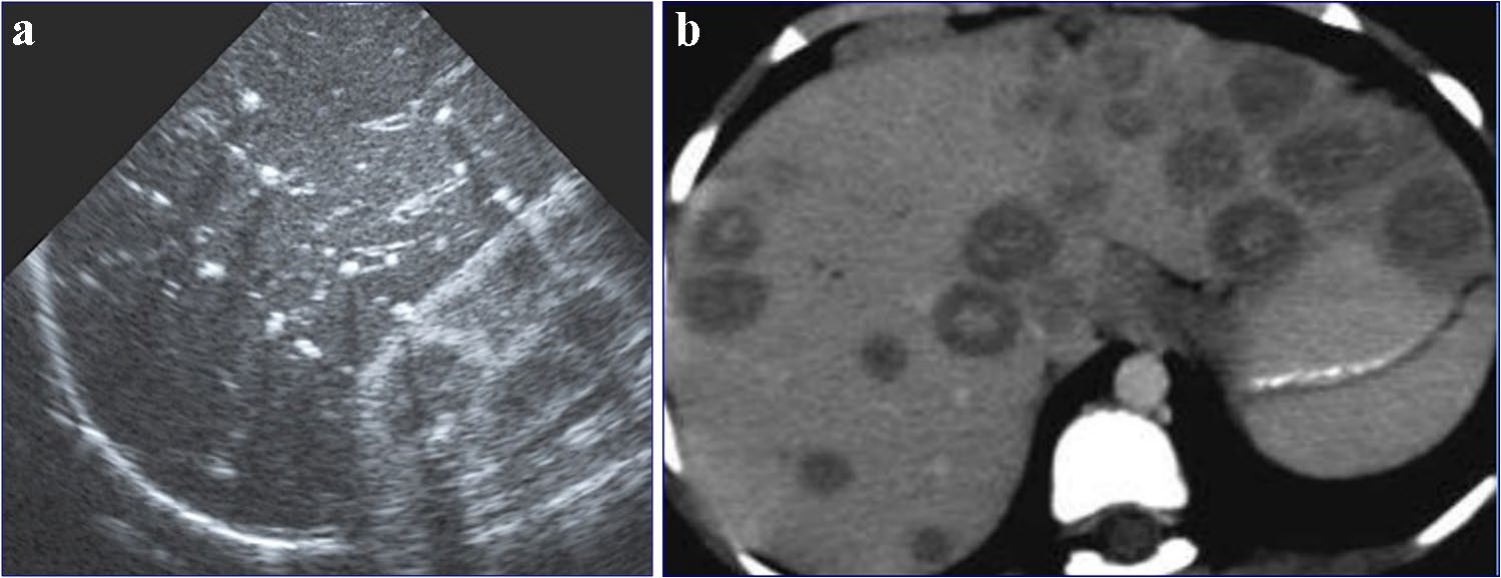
Hepatic tuberculosis (TB) in two children. a A transverse abdominal ultrasound in a 3-year-old boy reveals multiple small (3–5 mm) hyperechoic nodules, some of which exhibit posterior shadowing compatible with micronodular hepatic TB. b An axial contrast-enhanced computed tomography  abdominal image in a 7-year-old girl shows multiple round nodules measuring more than 10 mm. The lesions are low in attenuation with some showing central hyperattenuation. Some of the lesions in the left hepatic lobe coalesce and appear mass-like
